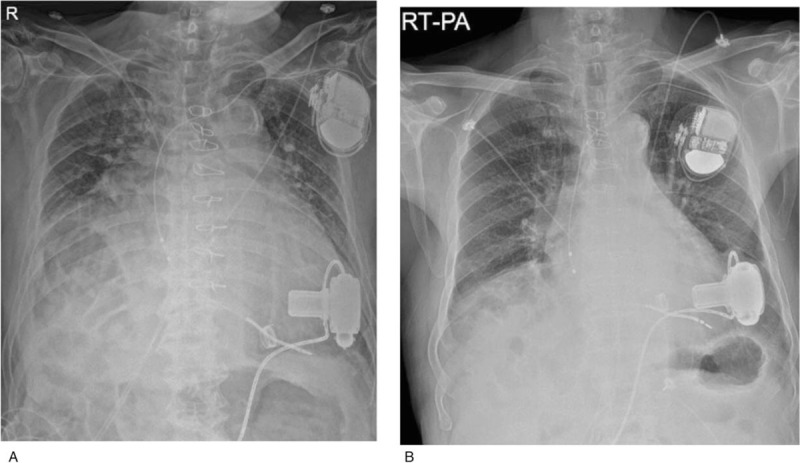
Chest radiography. (A) pre-RFCA shows bilateral haziness and pulmonary edema (B) Image before discharge after successful RFCA shows improved pulmonary edema and lung condition. RFCA = radiofrequency catheter ablation.
